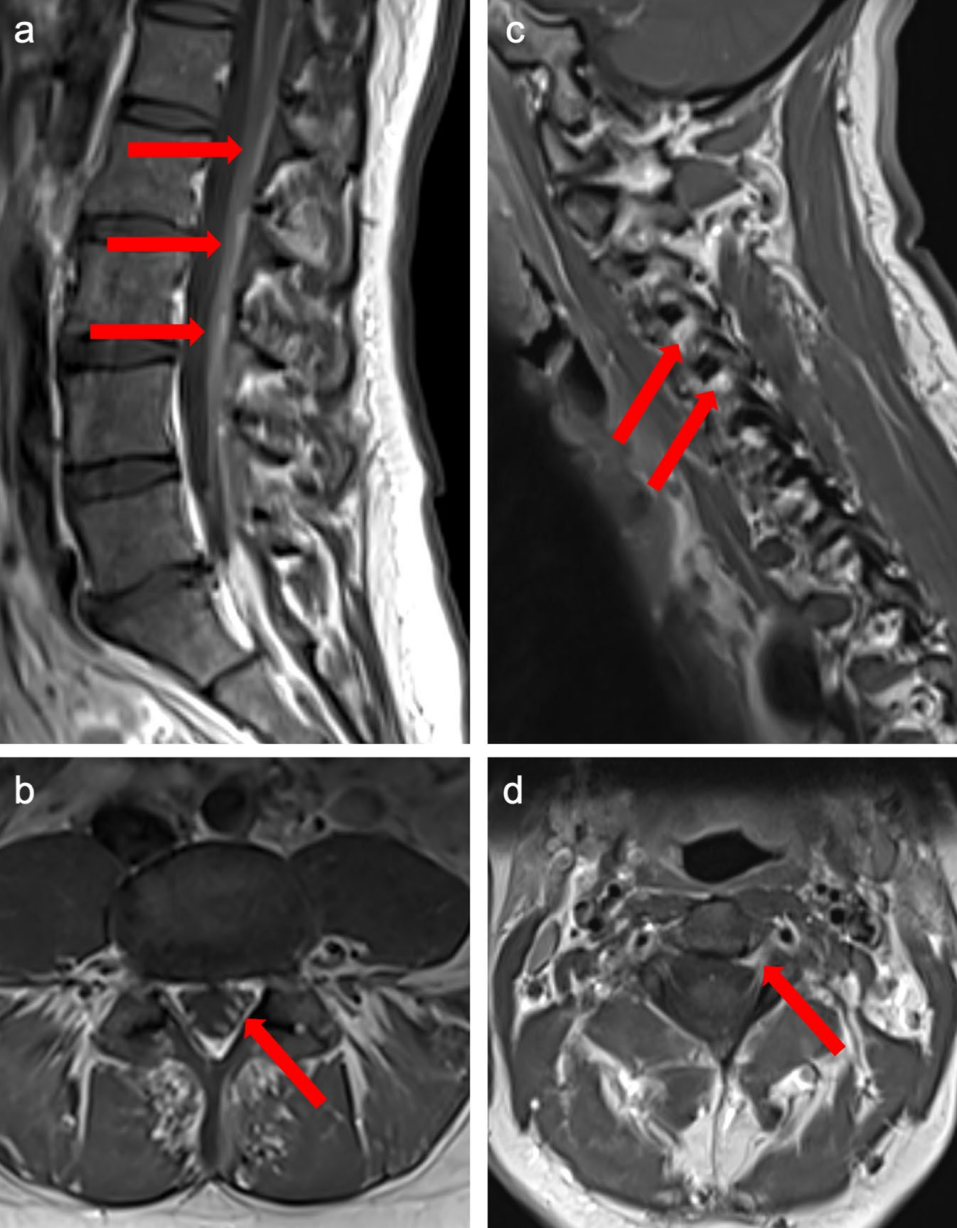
MRI findings of a patient with *SOD1*-ALS during treatment with tofersen. Red arrows indicate contrast enhancement. a Sagittal view of the lumbar spine. b Transversal view of the lumbar spine. c Sagittal view of the cervical spine. d Transversal view of the cervical spine. *SOD1*-*ALS*
